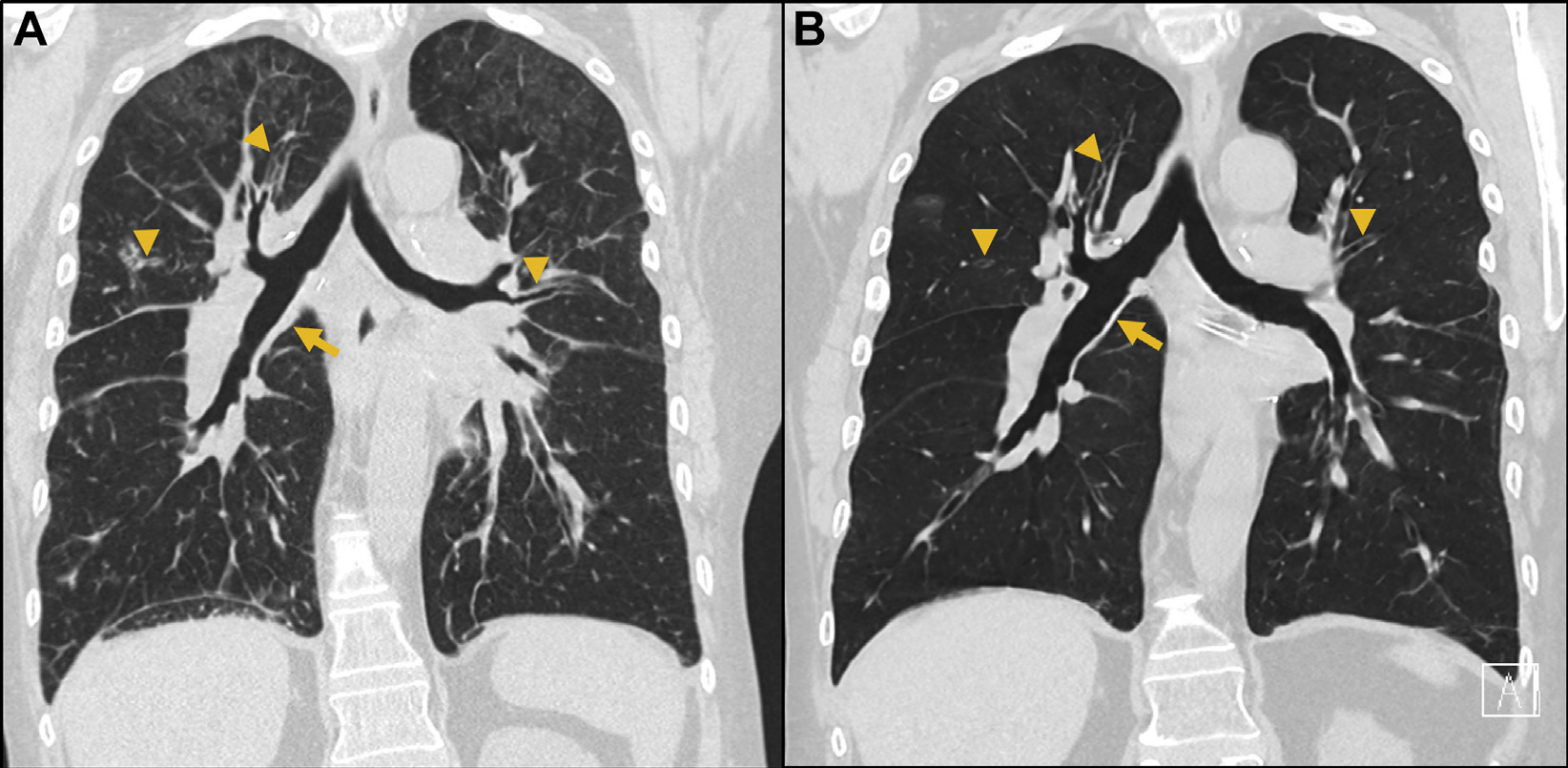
A, HRCT chest coronal reconstruction (22 months after lung transplantation for Sweet’s syndrome), highlighting diffuse small and large airway wall thickening involving the bronchus intermedius (arrow) and bilateral segmental airways (arrowheads). Multifocal and confluent ground-glass infiltrates are best visualized in the bilateral upper lobes. B, HRCT chest coronal reconstruction (23 months after lung transplantation for Sweet’s syndrome), highlighting the relatively rapid resolution of small and large airway wall thickening involving the bronchus intermedius (arrow) and bilateral segmental airways (arrowheads) after augmented corticosteroid therapy. In addition, the upper lobe predominant alveolar infiltrates demonstrate near resolution. HRCT = high-resolution CT.
